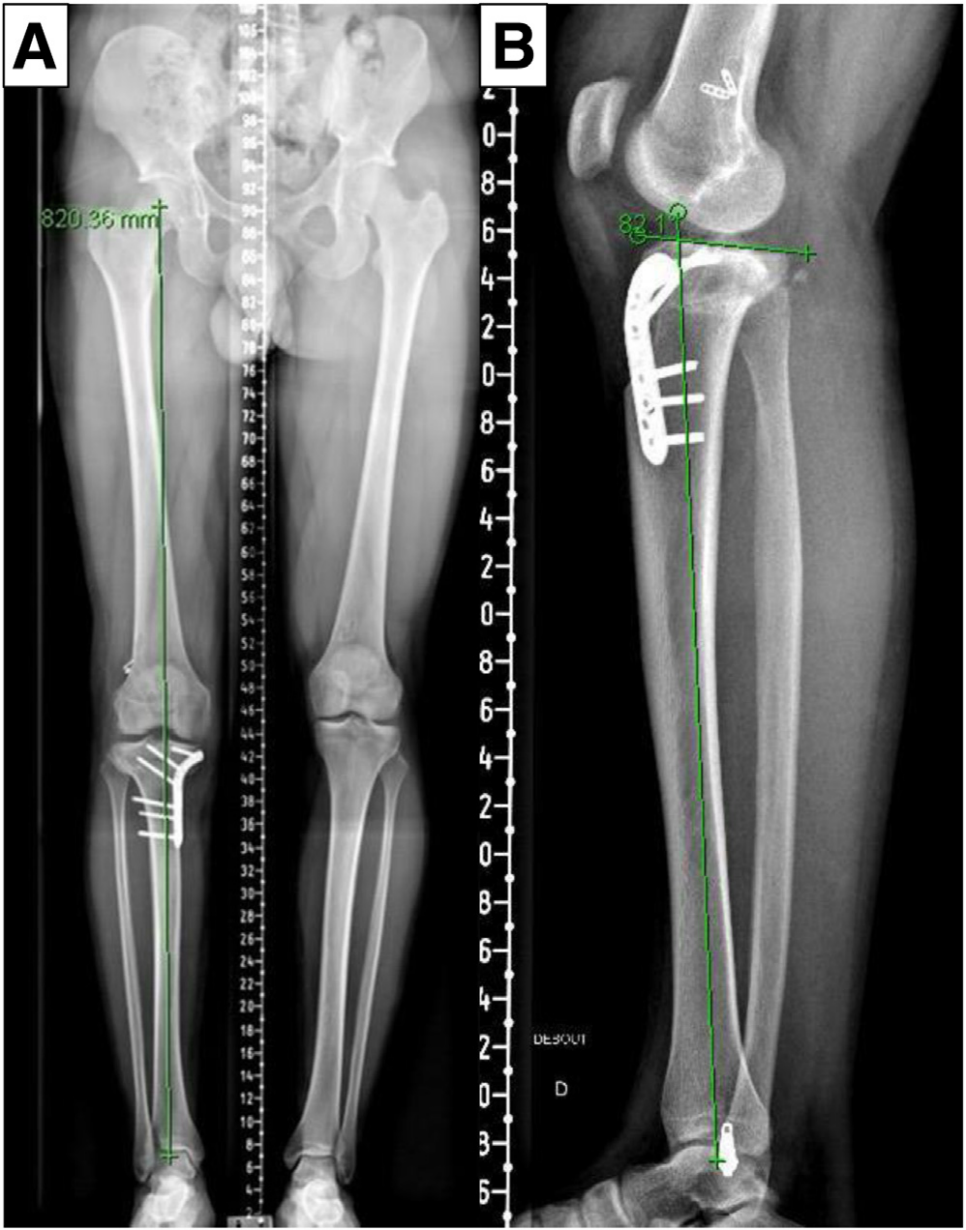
Postoperative plain radiograph. (A) Coronal long-leg radiograph shows correction to neutral alignment after the osteotomy. (B) Posterior tibial slope is reduced to 8° in the lateral radiograph.
